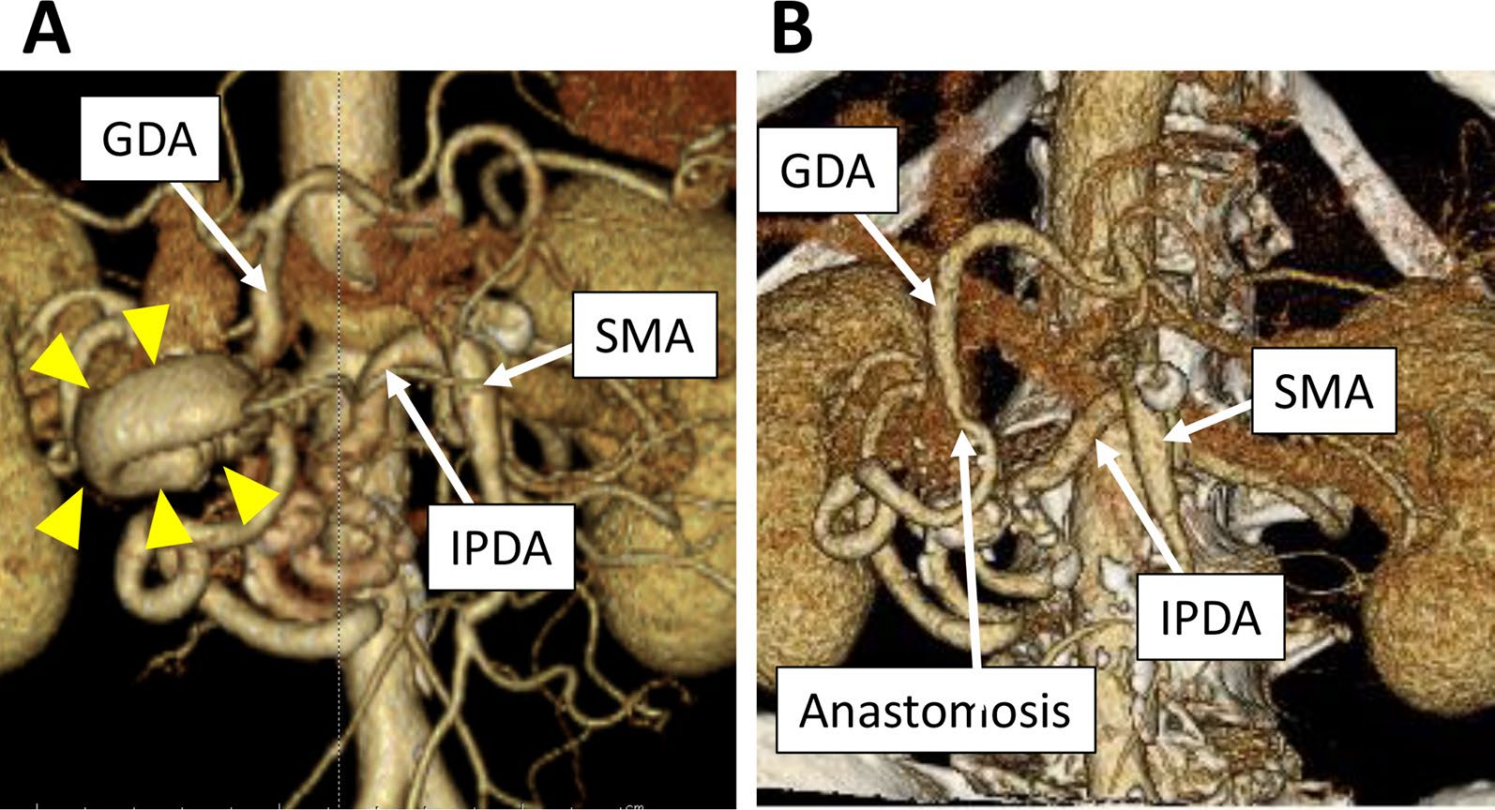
CT finding of the pancreatic arterial arcade in Patient 2. A Preoperative 3D-CT. The arrowheads indicate the pancreatic artery aneurysm. B 3D-CT 1 year and 8 months after the operation. *GDA* gastroduodenal artery, *IPDA* inferior pancreaticoduodenal artery, *SMA* superior mesenteric artery
